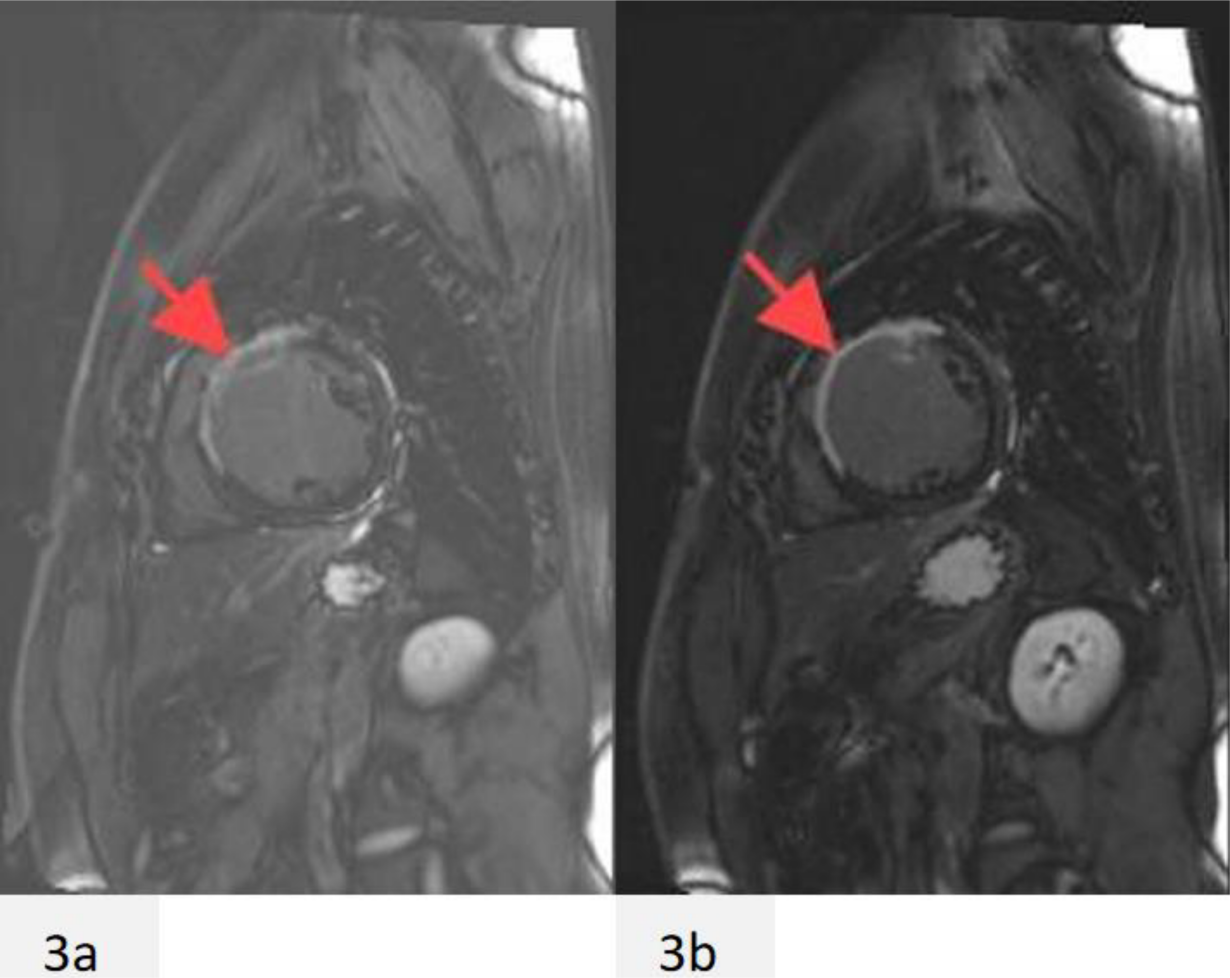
The patient underwent cardiac magnetic resonance imaging during their first admission (a,b). The area indicated by the arrow in the figure represents cardiac scars or fibrosis.
